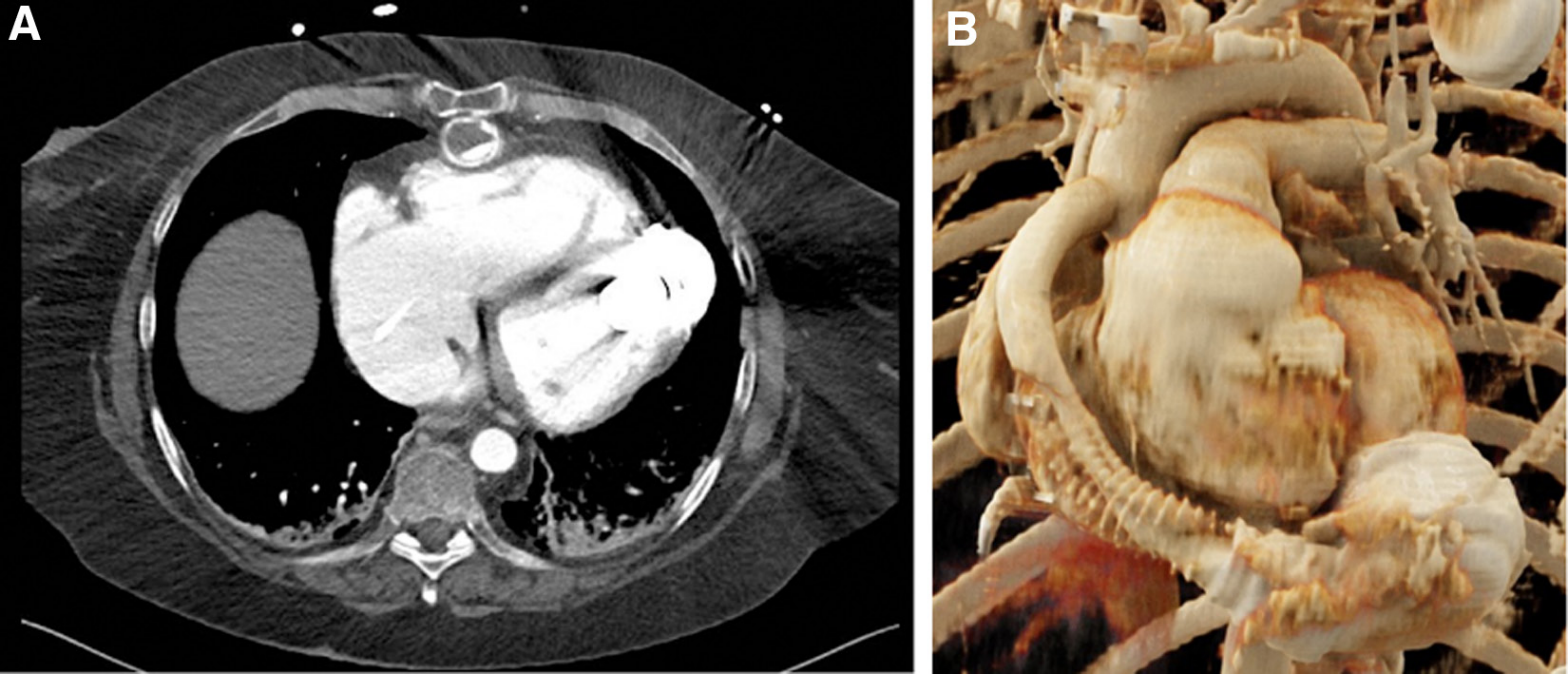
Imaging demonstrating proximal outflow graft obstruction in a 63 year old female with cardiogenic shock. A: Computed tomography angiography revealing a filling defect of uncertain etiology in the proximal outflow graft at the level of the Bend Relief. B: Three-dimensional reconstructions with the appearance of mechanical obstruction secondary to compression external to the graft. Graft torsion and/or thrombosis could not be excluded on these images.
